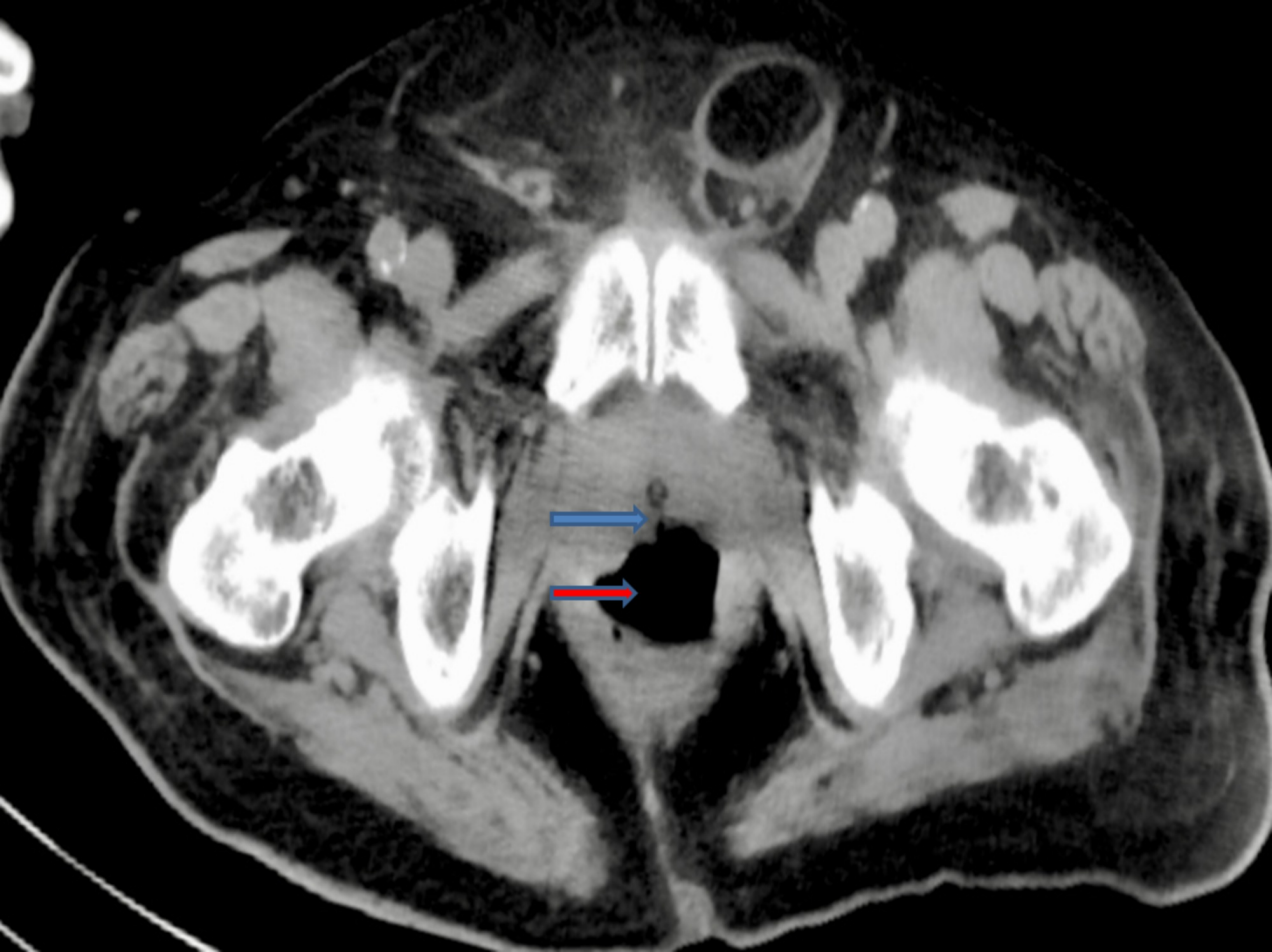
Computed tomography (CT) abdomen and pelvis revealed the rectourethral fistula Blue arrow shows the rectourethral fistula Red arrow shows the rectum
